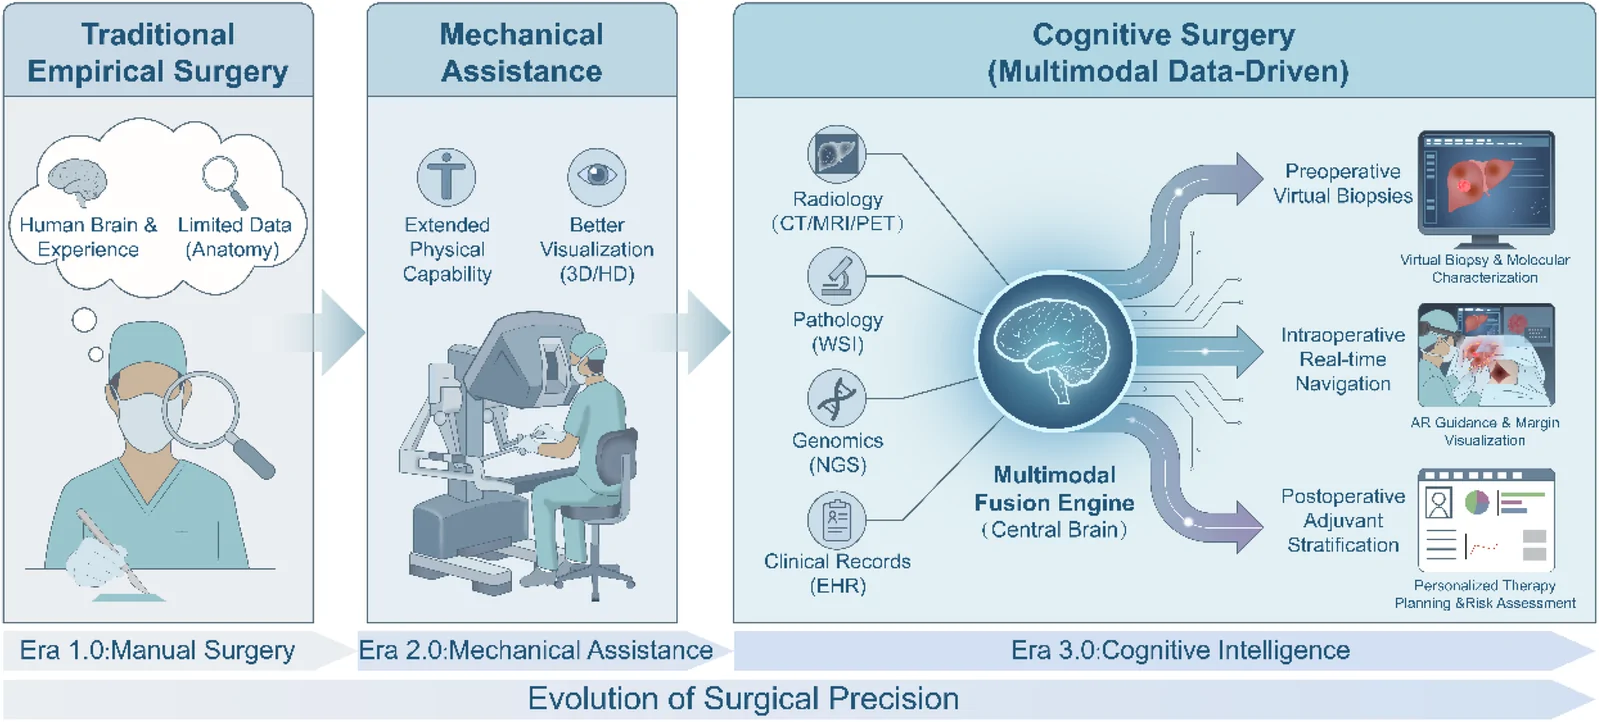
The evolution of surgical precision: From mechanical assistance to cognitive intelligence. Era 1.0 (Manual Surgery): Clinical decisions were based on the surgeon's personal experience and limited anatomical information that was often fragmented. Era 2.0 (Machine-Assisted Surgery): a technological transition driven by robotic surgical platforms and high-definition or three-dimensional visualization systems. Era 3.0 (Cognitive Intelligence): an emerging data-oriented paradigm focused on multimodal information integration. In this framework, a multimodal fusion system combines previously separated clinical data sources, including medical imaging (CT/MRI/PET), pathological whole-slide images (WSI), genomic information from next-generation sequencing (NGS), and electronic health records (EHR).
